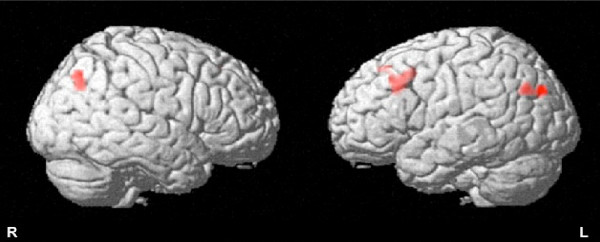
Spatial distribution of functional brain MRI activation.
